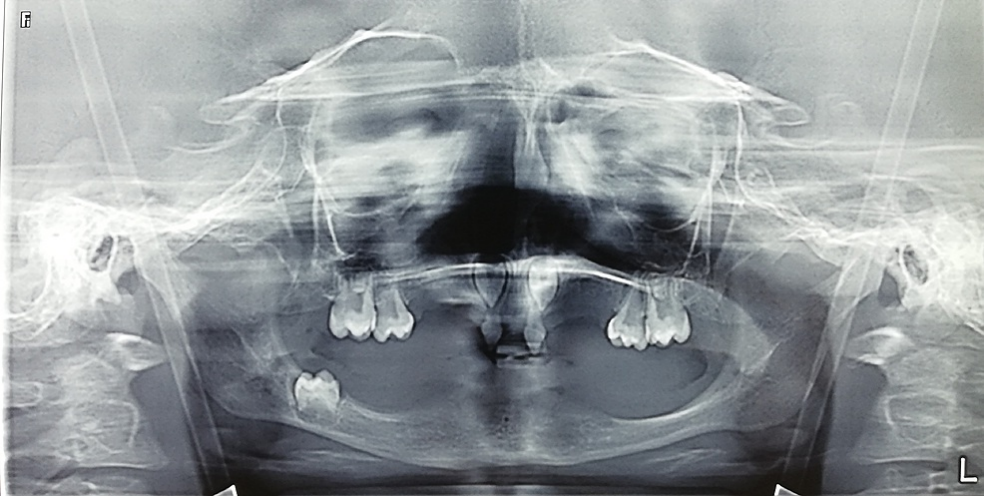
Orthopantomogram shows the absence of teeth in the mandible.
